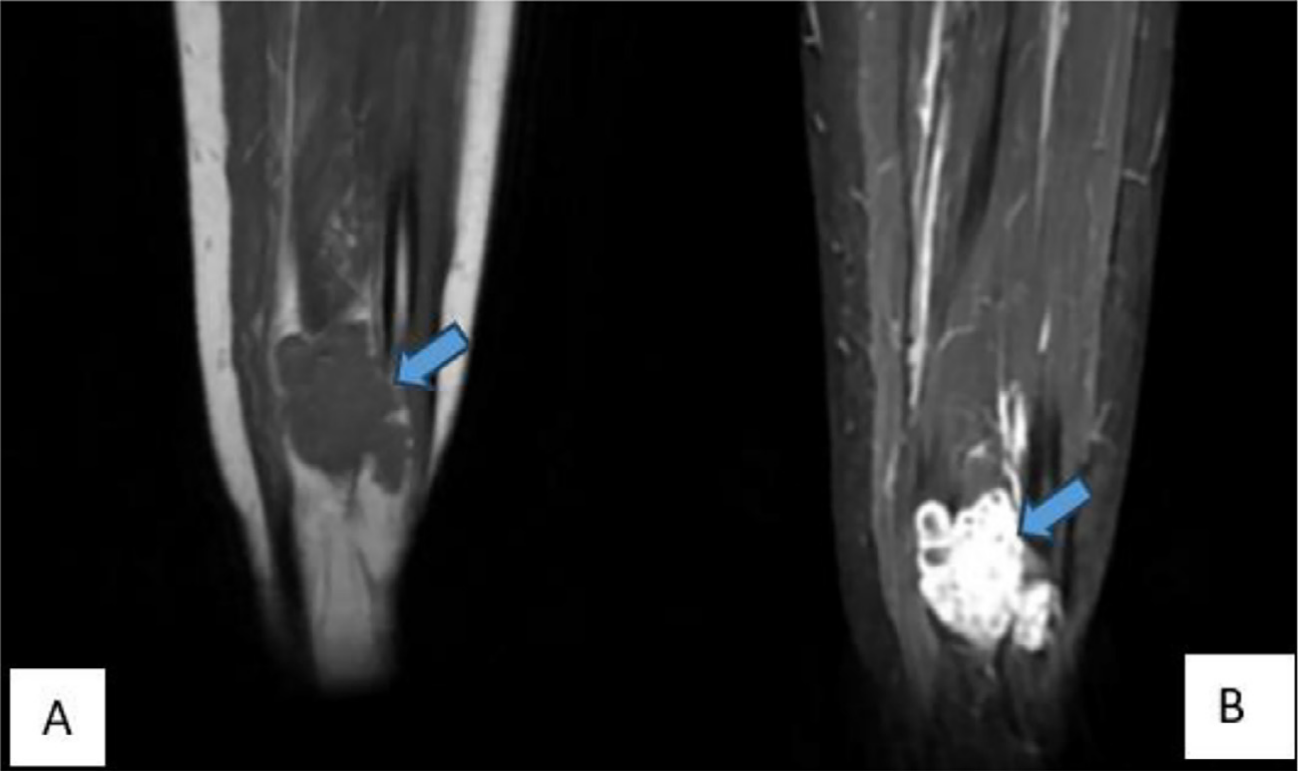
(A) Coronal T1-weighted MRI and (B) coronal STIR sequence demonstrating a well-defined, macro-lobulated intramuscular lesion within the flexor hallucis longus muscle. The lesion appears hypointense on T1-weighted images and markedly hyperintense on STIR sequences.
